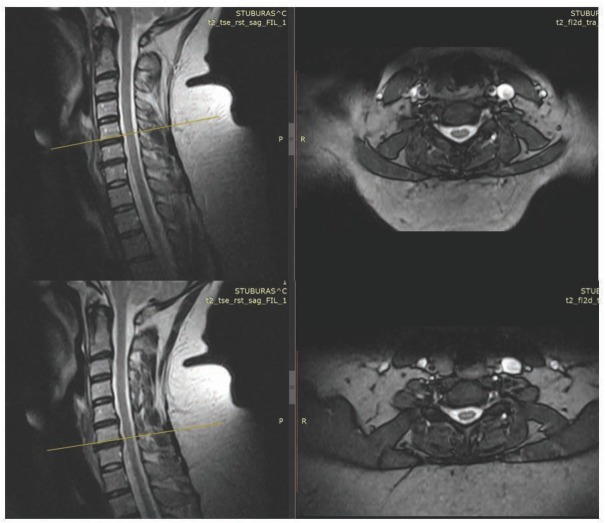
Magnetic resonance imaging of the cervical spine shows intervertebral hernias in C5-C6, C6-C7, and cervical spinal stenosis.
